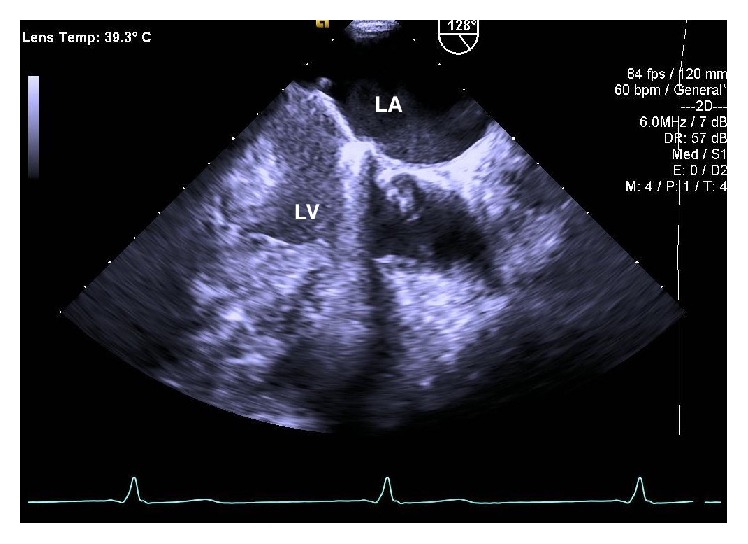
Repeat transesophageal echocardiogram repeated after six weeks of antibiotic treatment showing resolution of the vegetation. LA: left atrium; LV: left ventricle.
